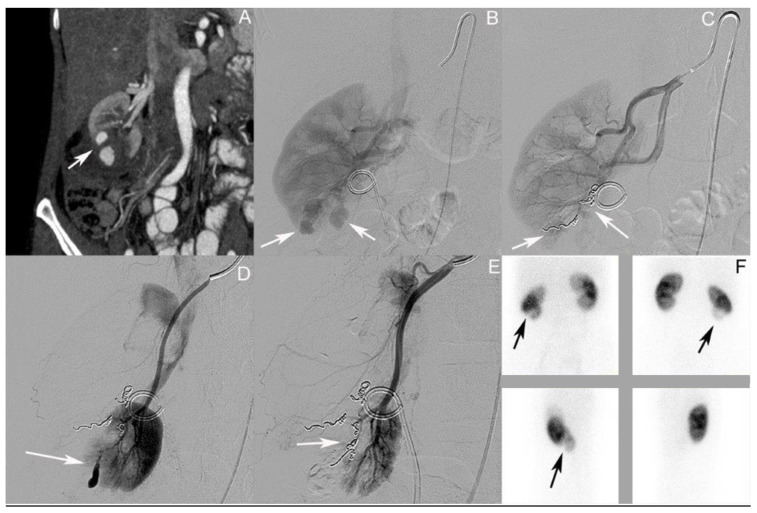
Selected imaging of case III. (A) Coronary contrast-enhanced computed tomography (CT) scan of the abdomen; the arrow shows several up to 20 mm in size AV fistulas/pseudoaneurysms in the ruptured parenchyma of the lower pole of the right kidney. (B) Angiography of the right kidney with Double-J ureteral stent in situ; the arrow shows the AV fistulas. (C) Angiography post coil embolization; the arrow shows the coils in situ in the lower pole of the right kidney. (D) Repeat angiography showing persistent AV fistula (arrow) post second coil embolization; the arrow shows the coils in situ in the lower pole of the left kidney. (E) Angiography post second coil embolization; the arrow shows the coils in situ in the lower pole of the right kidney. (F) Technetium-99 m dimercaptosuccinic acid (DMSA) renal scintigraphy showing no tracer accumulation in the lower kidney pole on the right side (arrow); contribution left/right kidney of 61%:39%.
